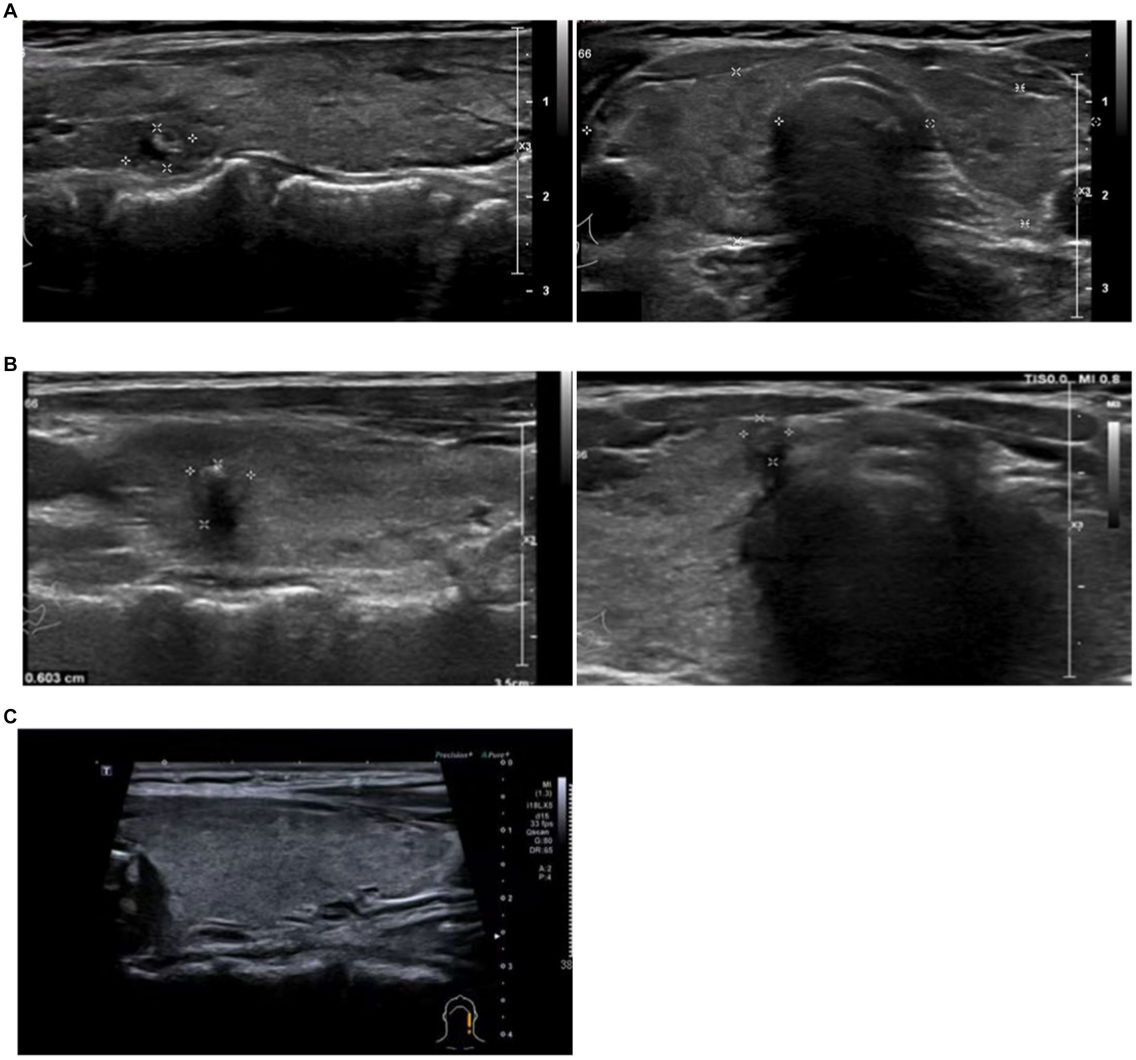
Thyroid ultrasonography. Thyroid ultrasonography of case 1 (A), Right lobe: 20 × 13 mm. Left lobe: 17 × 9 mm. Bilateral thyroid echo thickening and bilateral thyroid nodules (label). Case 2 (B), Right lobe: 20 × 20 mm. Left lobe: 21 × 19 mm. Bilateral thyroid echo thickening and bilateral thyroid nodules (label). Case 5 (C), bilateral thyroid echo thickening.
